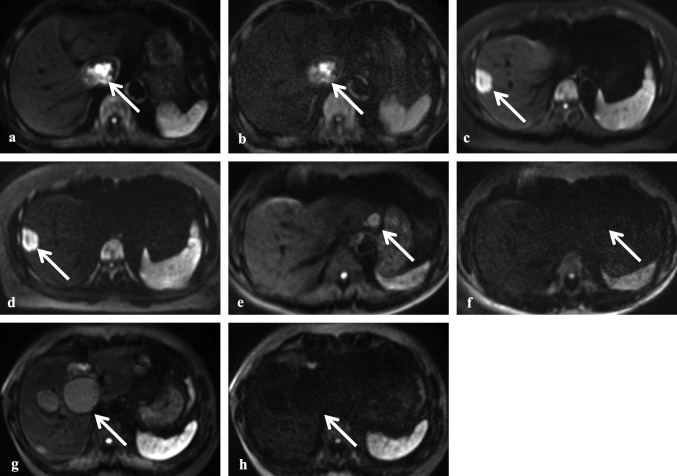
Representative diffusion-weighted imaging (DWI) appearance of four different hepatic mass types at b-values of 1000 and 3000 s/mm^2^ Representative liver DWI images of four different hepatic mass types at b-values of 1000 and 3000 s/mm^2^: a, b: A 78-year-old man with hepatocellular carcinoma (HCC); c, d: A 47-year-old woman with hepatic metastasis from rectal carcinoma; e, f: A 57-year-old man with hepatic hemangioma; g, h: A 66-year-old woman with a hepatic cyst. a, c, e, g show liver DWI at a b-value of 1000 s/mm^2^, while b, d, f, h show DWI at a b-value of 3000 s/mm^2^. The HCC (a, b) and hepatic metastasis (c, d) exhibit high signal intensity (SI) similar to or greater than that of the spleen at both b-values. In contrast, the hepatic hemangioma (e, f) and cyst (g, h) are visible with SI similar to or less than that of the spleen at 1000 s/mm^2^ (e, g) but are not visible at 3000 s/mm^2^ (f, h).
